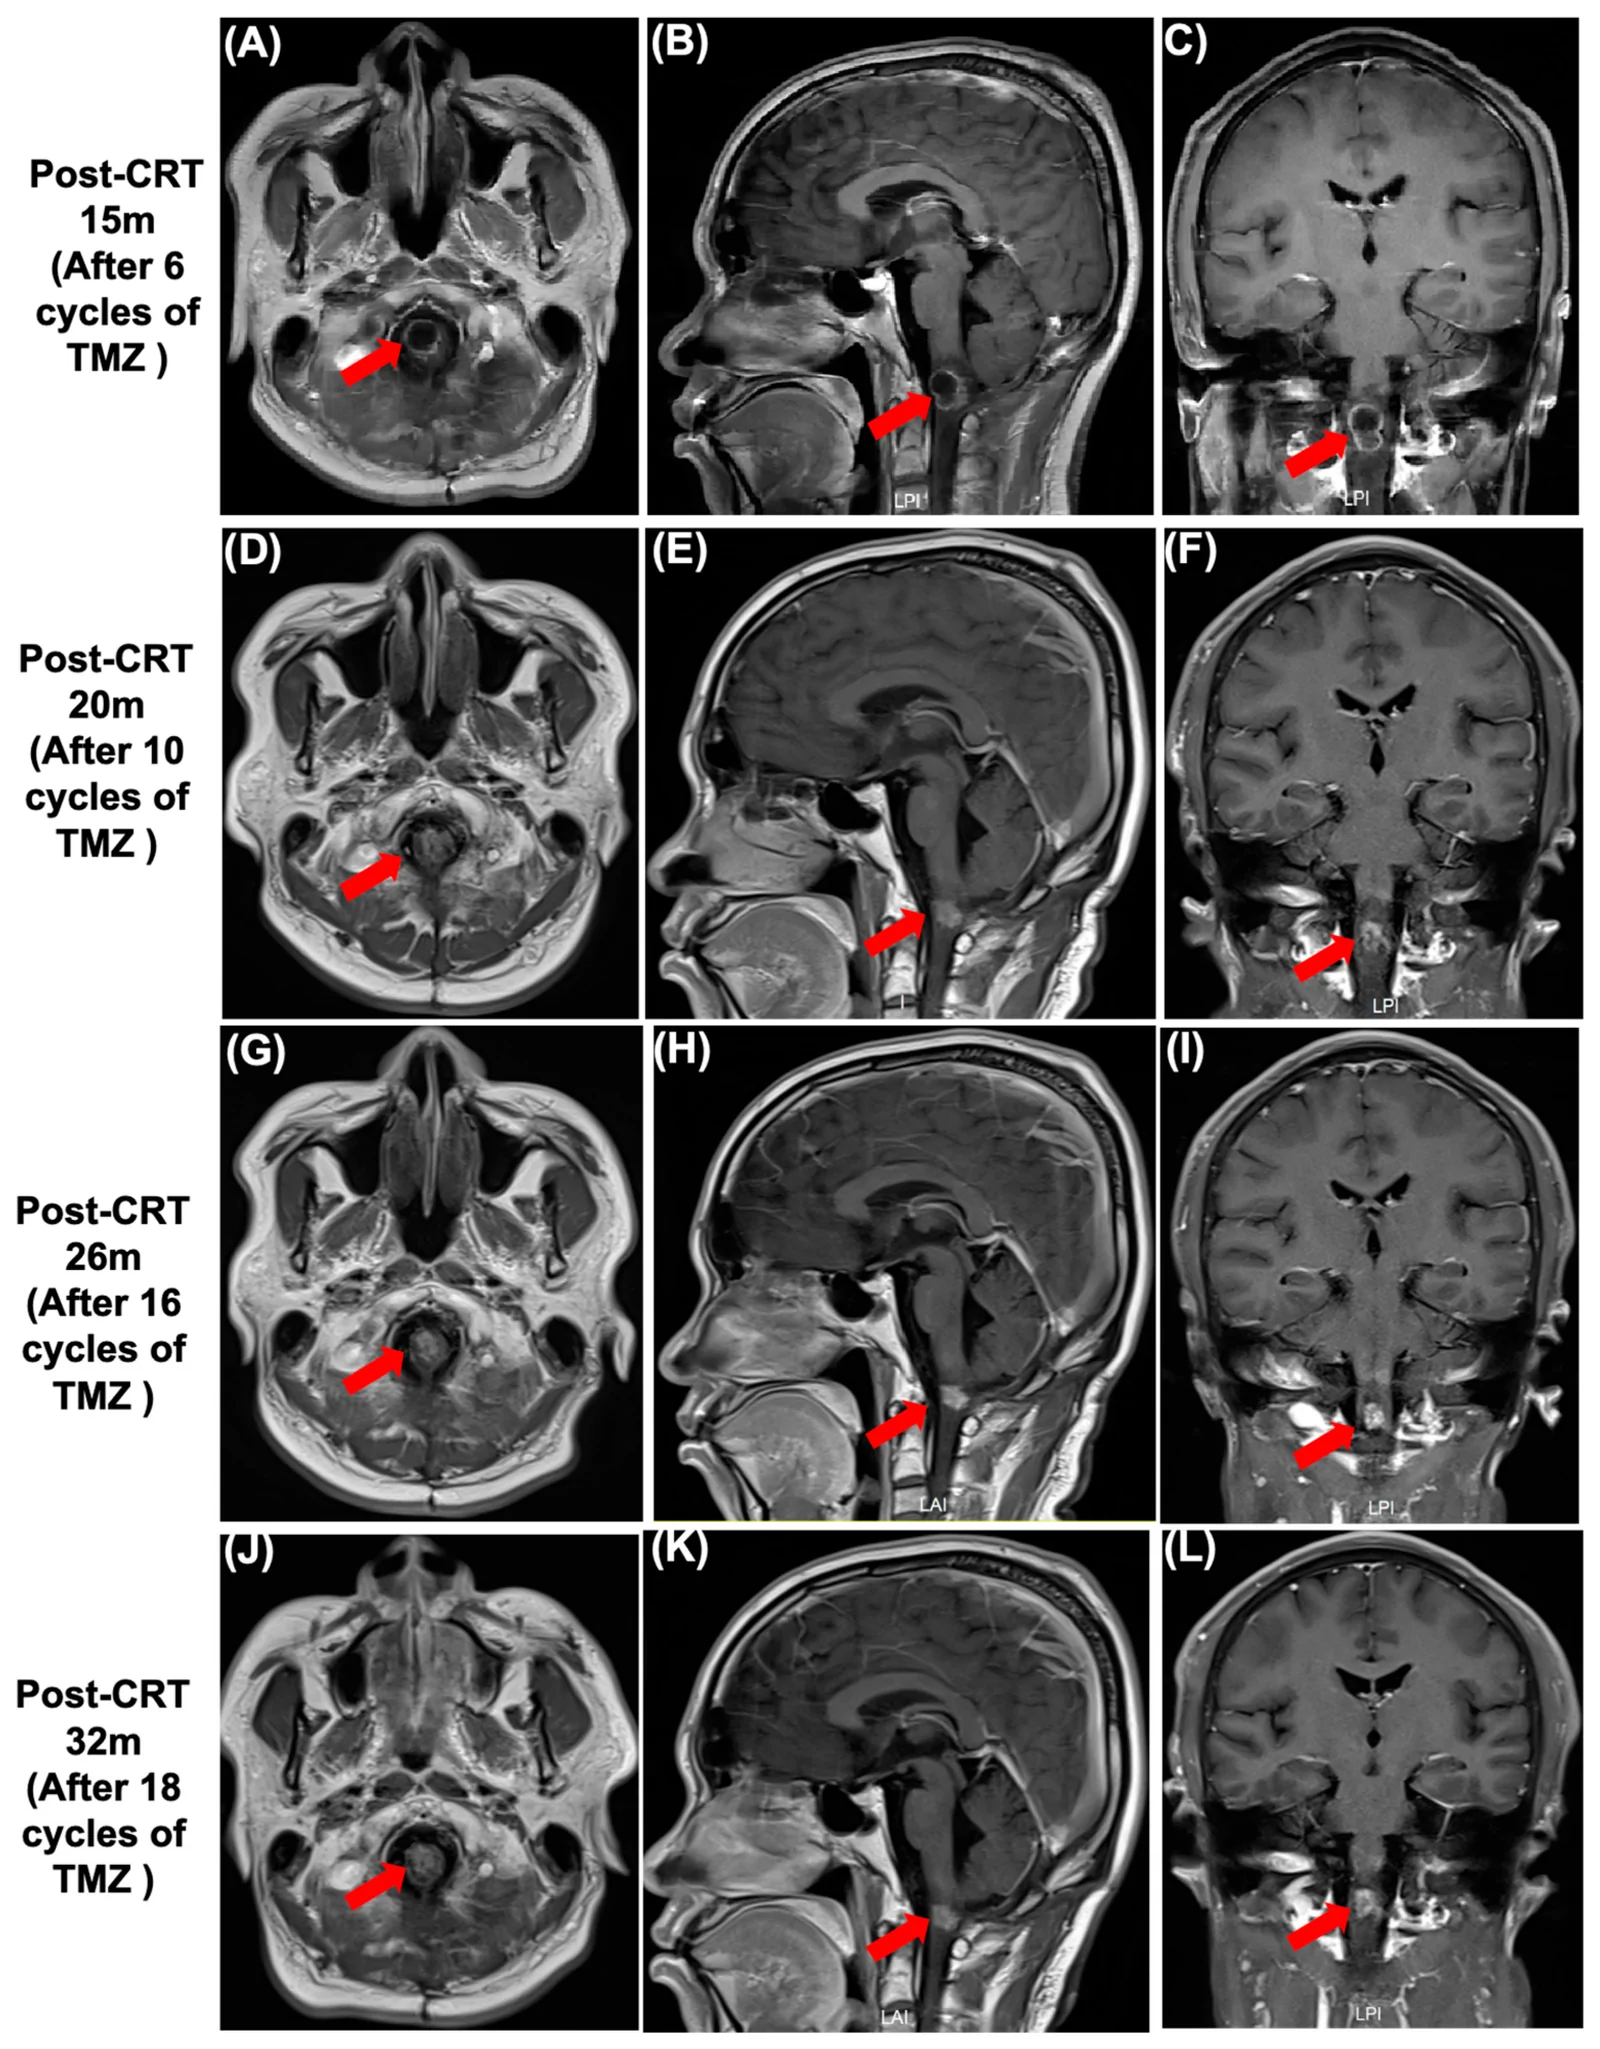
Cranial MRI images demonstrate brainstem lesions at different time points after TMZ maintenance chemotherapy. (A–C) Axial, sagittal, and coronal views of cranial MRI show a tumor mass measuring 15 mm × 13 mm × 18 mm 15 months after CRT; (D–F) axial, sagittal, and coronal views of cranial MRI show a tumor mass measuring 14 mm × 11 mm × 10 mm 20 months after CRT; (G–I) axial, sagittal, and coronal views of cranial MRI show a tumor mass measuring 12 mm × 11 mm × 10.5 mm 26 months after CRT; and (J–L) axial, sagittal, and coronal views of cranial MRI show a tumor mass measuring 12 mm × 11 mm × 11 mm 32 months after CRT. The position pointed by the arrow is the site of the lesion.
